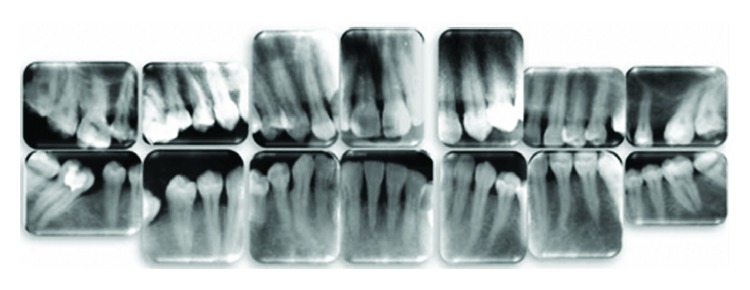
Final periapical radiographs 2 years post treatment.
